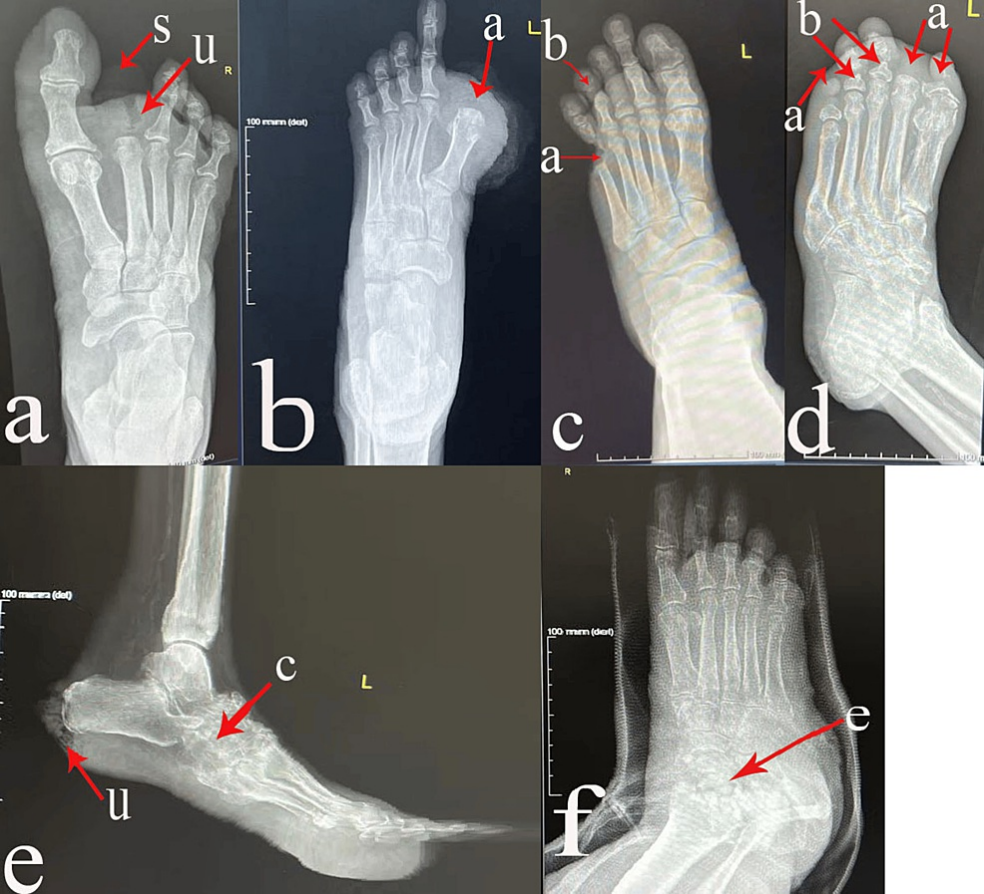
Radiographs of the foot and ankle region of some studied cases a- Forefoot swelling and ulceration (u). Second toe phalangeal bone (s) amputation; b- Hallux amputation (a); c- Deep ulceration accompanied by soft tissue loss and bone loss at the fifth metatarsophalangeal joint (a). There is extensive refraction, erosion, and resorption of the middle and proximal phalanges of the fourth toe (b); d- Hallux, second, and fifth toes were amputated (a). An old fracture of the proximal phalangeal bones of the third and fourth toes (b); e- An ulcer and gas lucencies (u) near the heel region suggest infection or gangrene. The intratarsal and tarsometatarsal joints were affected by erosions, fragmentations, deformities, and Charcot's arthropathy (c); f- The hindfoot is rarefacted, eroded, and fragmented (e).
